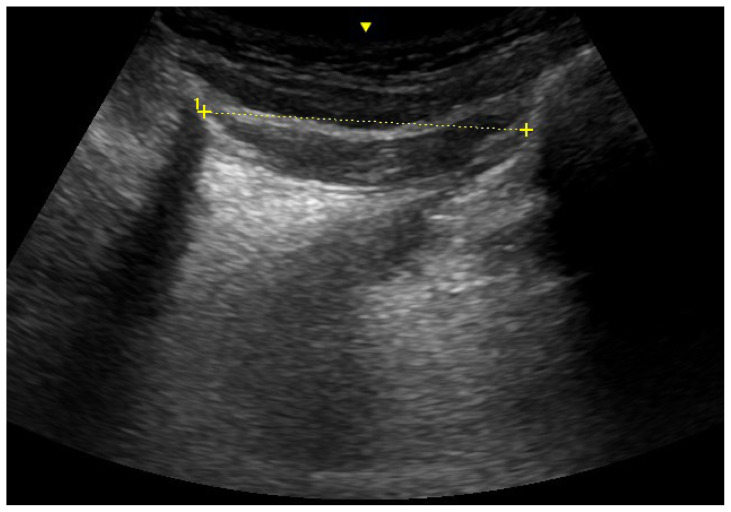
Sonographic measurement of hyomental distance.
